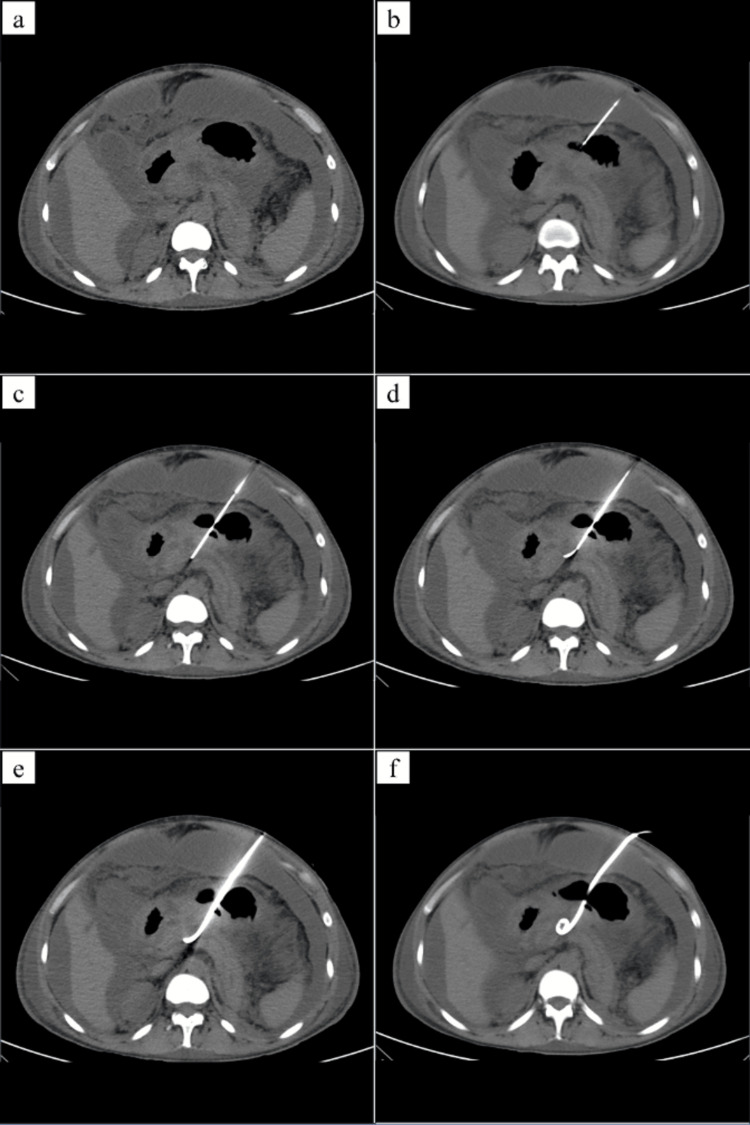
Steps of CT-guided transgastric drain placement (a) An ill-defined, walled-off pancreatic necrosis in the lesser sac. (b) Placement of an 18-G needle across the anterior wall of the stomach. (c) Puncturing both the anterior and posterior walls of the stomach. (d) Passage of 0.038-inch stiff wire through the needle. (e) Dilatation of the tract using 10 Fr Coon’s dilator (f) Placement of 10 Fr locking loop pigtail catheter
